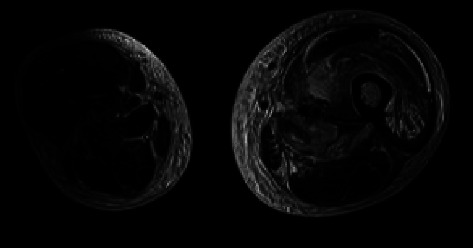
T1-weighted MRI (axial) showing muscular oedema of left thigh—affecting rectus femoris, vastus medialis, vastus lateralis, and adductor tendons including the adductor longus, adductor brevis, adductor magnus, adductor gracilis, and pectineus muscles.
